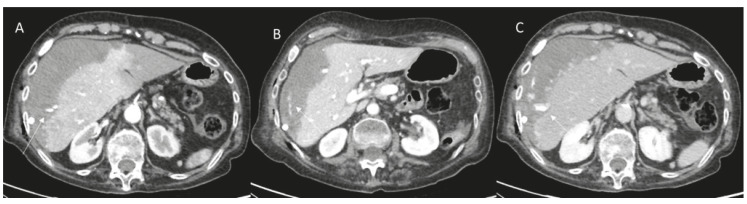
Woman 65 year at 7-day follow-up after RFA of liver metastases. CT assessment ((A): arterial phase; (B): portal phase and (C): late phase): active bleeding (arrow).
